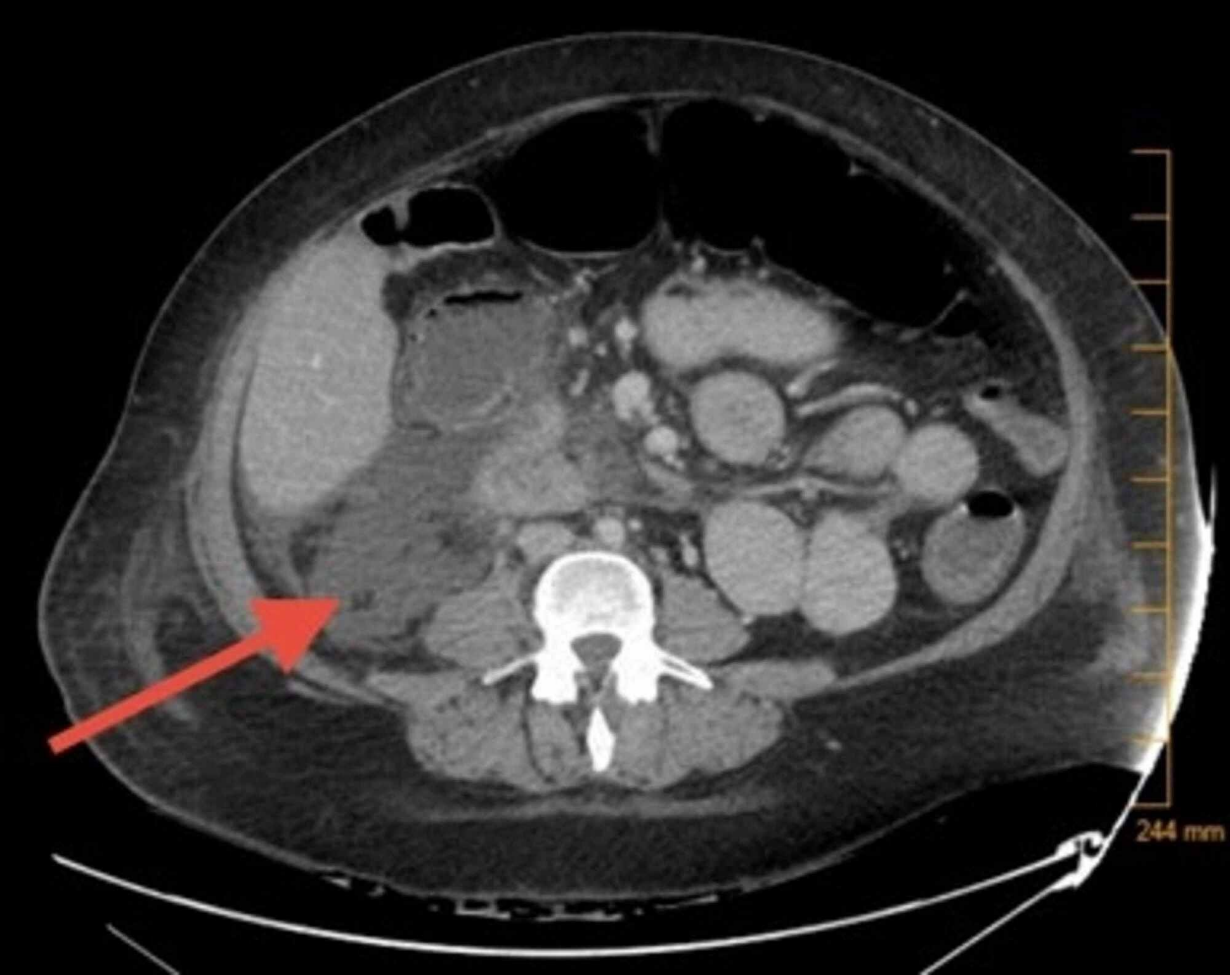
Abdominal CT scan demonstrating a right perinephric fluid collection as indicated by the arrow CT: Computed tomography
